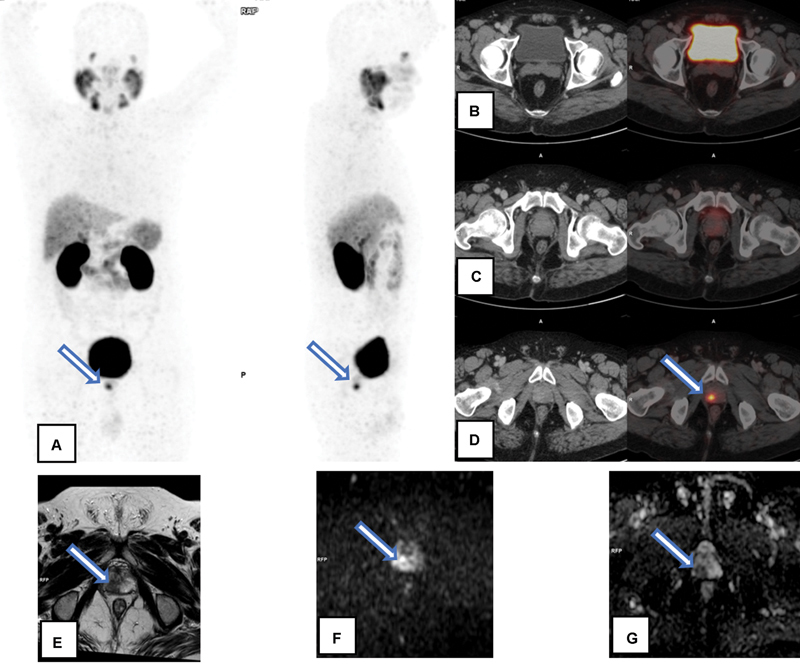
A 59-year-old patient with serum prostate-specific antigen—29.7 ng/mL, adenocarcinoma prostate with Gleason's score 3 + 3- Concordance between gallium-68 prostate-specific membrane antigen positron emission tomography-computed tomography (Ga-68 PSMA PET/CT) and multiparametric magnetic resonance imaging (mpMRI) in primary prostate lesion detection: Ga-68 PSMA PET/CT (
